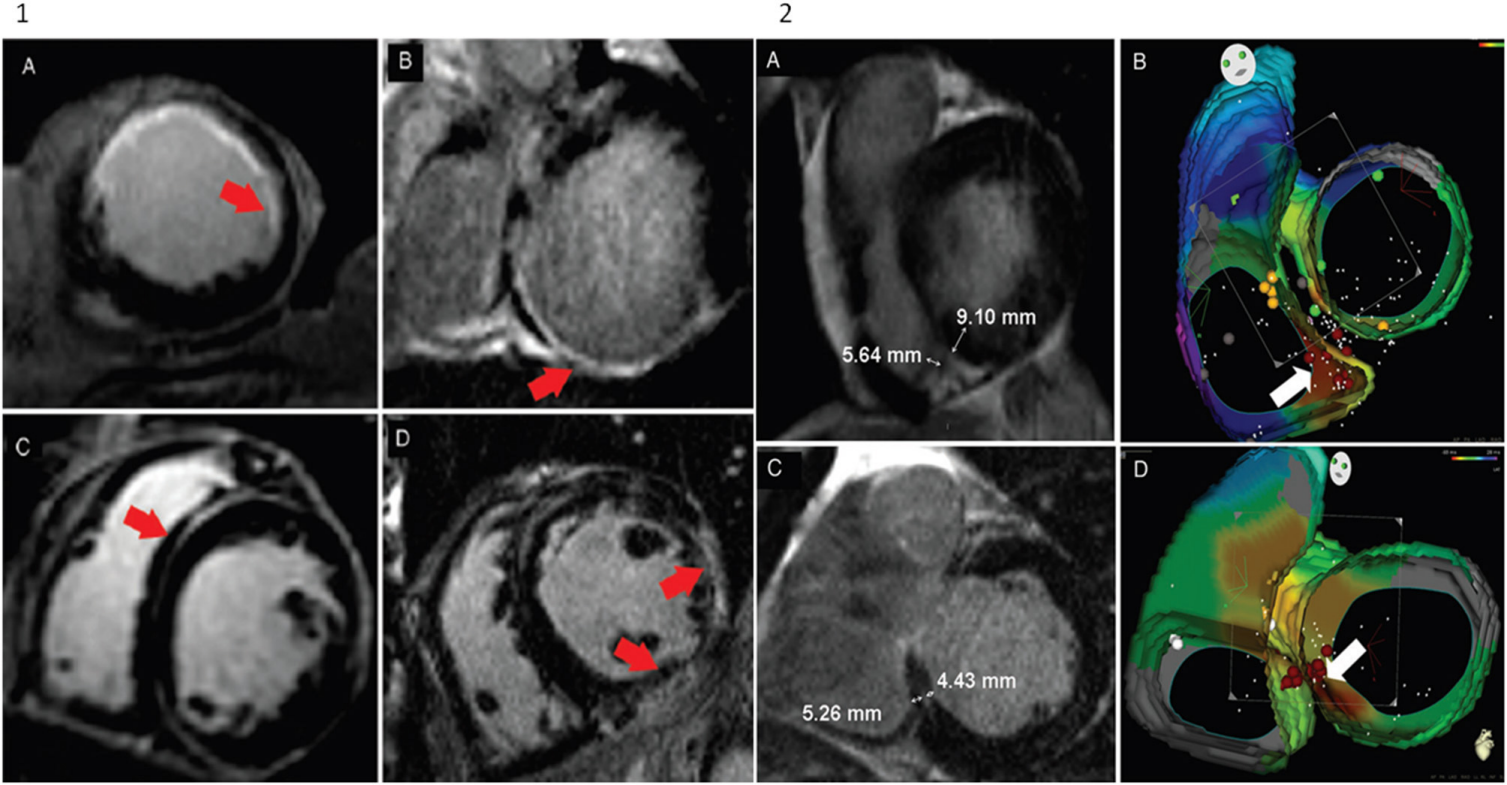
Adapted from Andreu et al. (29). Panel 1: Pattern distribution of hyperenhancement in cardiac magnetic resonance images. (A) Endocardial hyperenhancement in left ventricle the anterior wall. (B) Transmural hyperenhancement in the left ventricle posterior wall and endocardial hyperenhancement of the posteroseptal wall. (C) Mid-myocardial hyperenhancement in the anteroseptal wall. (D) Epicardial hyperenhancement in the left ventricle lateral wall. Panel 2: Cardiac magnetic resonance and electroanatomical map of two patients with mid-myocardial hyperenhancement at the successful ablation site. (A,B) Endocardial ablation of premature ventricular contractions originating from the right ventricle. (A) The distance to the boundary of the hyperenhancement region was shorter in the right ventricle than in the left ventricle. (B) Radiofrequency ablation of the right ventricle was performed. (C,D) Endocardial ablation of the left ventricle. (C) In this case, the distance to the boundary of the hyperenhancement region was shorter from the left ventricle than from the right ventricle. (D) A previous unsuccessful radiofrequency ablation was attempted in the right ventricle. After mapping, the left ventricle the maximum precocity of the left ventricle septum was obtained, and radiofrequency ablation eliminated premature ventricular contraction.
